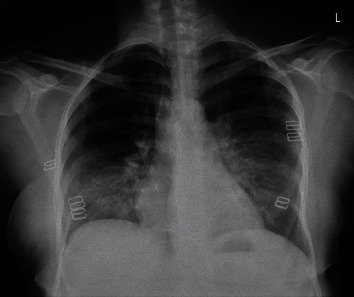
Brain CT scan showing no space occupying lesions nor hemorrhage in the parenchyma, a normal ventricular system with no hydrocephalus and no midline shift.
